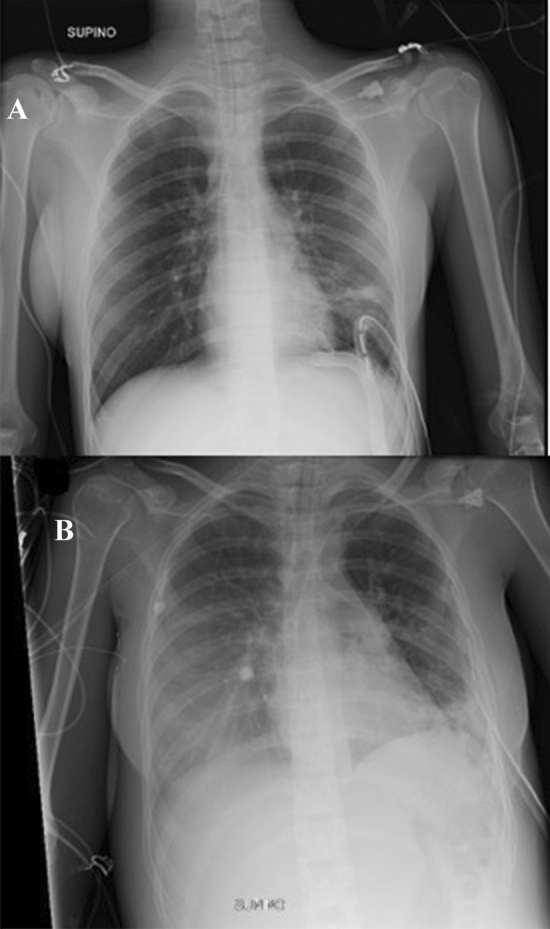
Chest X-ray soon after LVAD insertion (a) and after LVAD removal (b)
